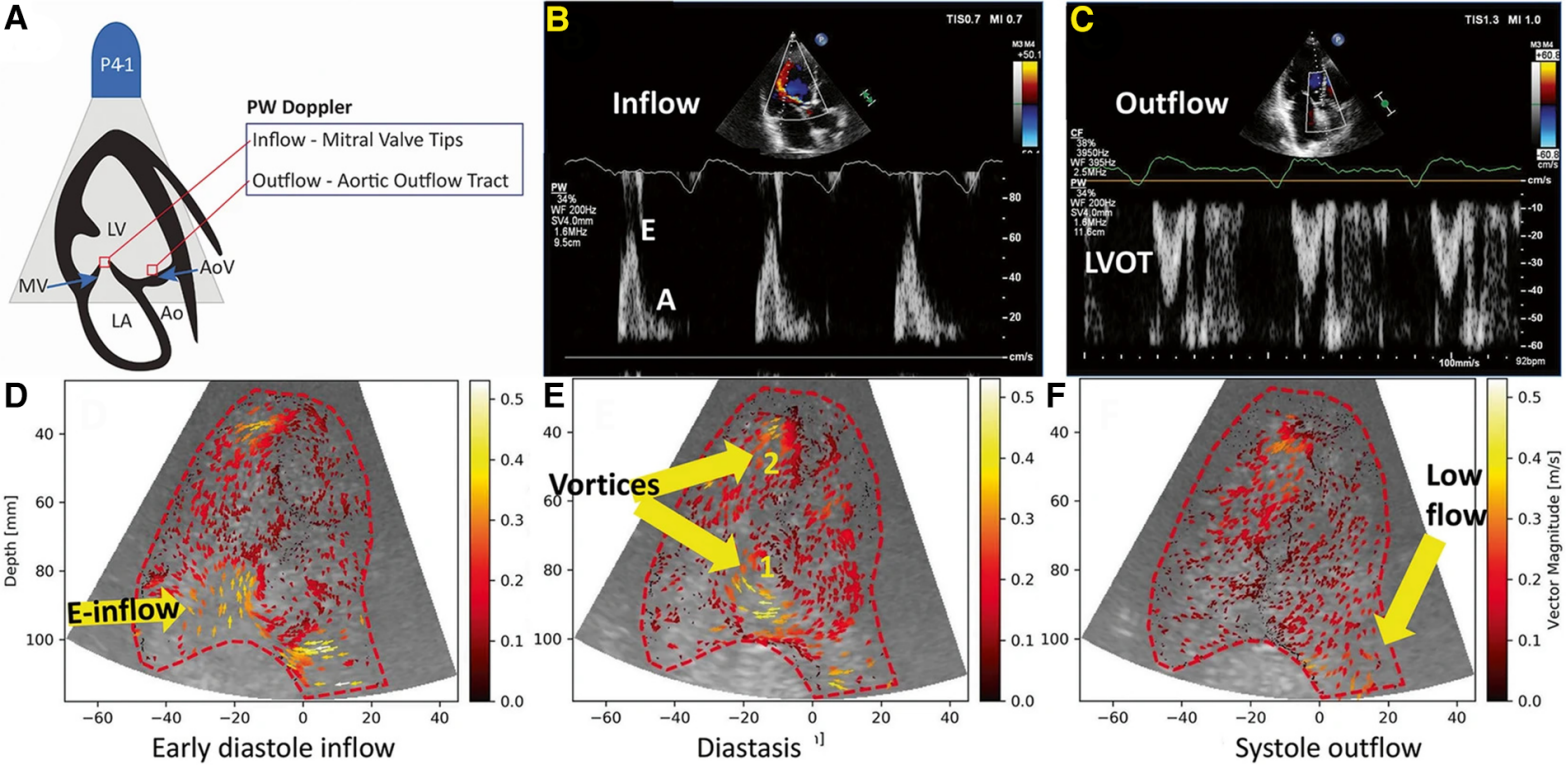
(A) Positioning of the Doppler and echo PIV interrogation points. (B,C) PW Doppler of inflow and outflow for a heart failure patient. (D–F) Results from echo PIV tracking flow in the same patient. This figure is adapted from Strachinaru et al. (2022, Figure 2), under a Creative Commons Attribution 4.0 International License (45).
